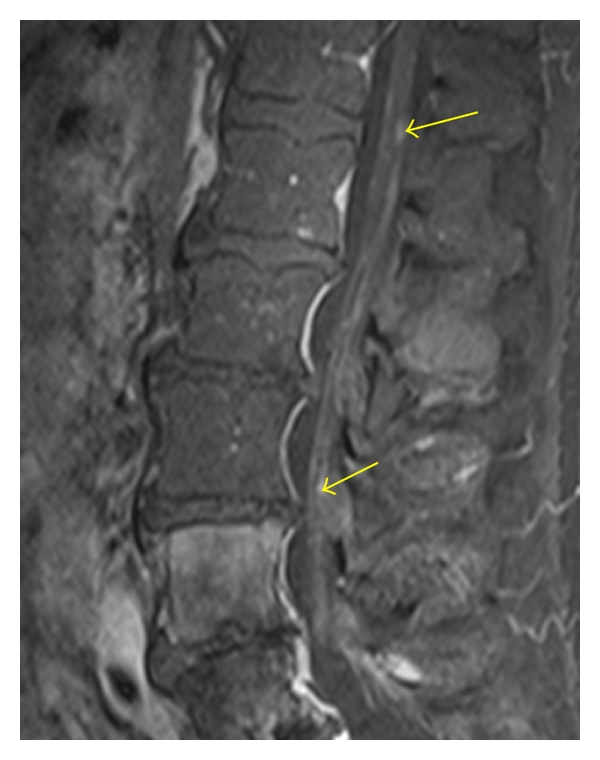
Sagittal contrast-enhanced T1-weighted MR image with fat saturation reveals multiple small enhancing lesions (yellow arrows) along the cauda equina, related to leptomeningeal carcinomatosis in a patient with breast cancer.
